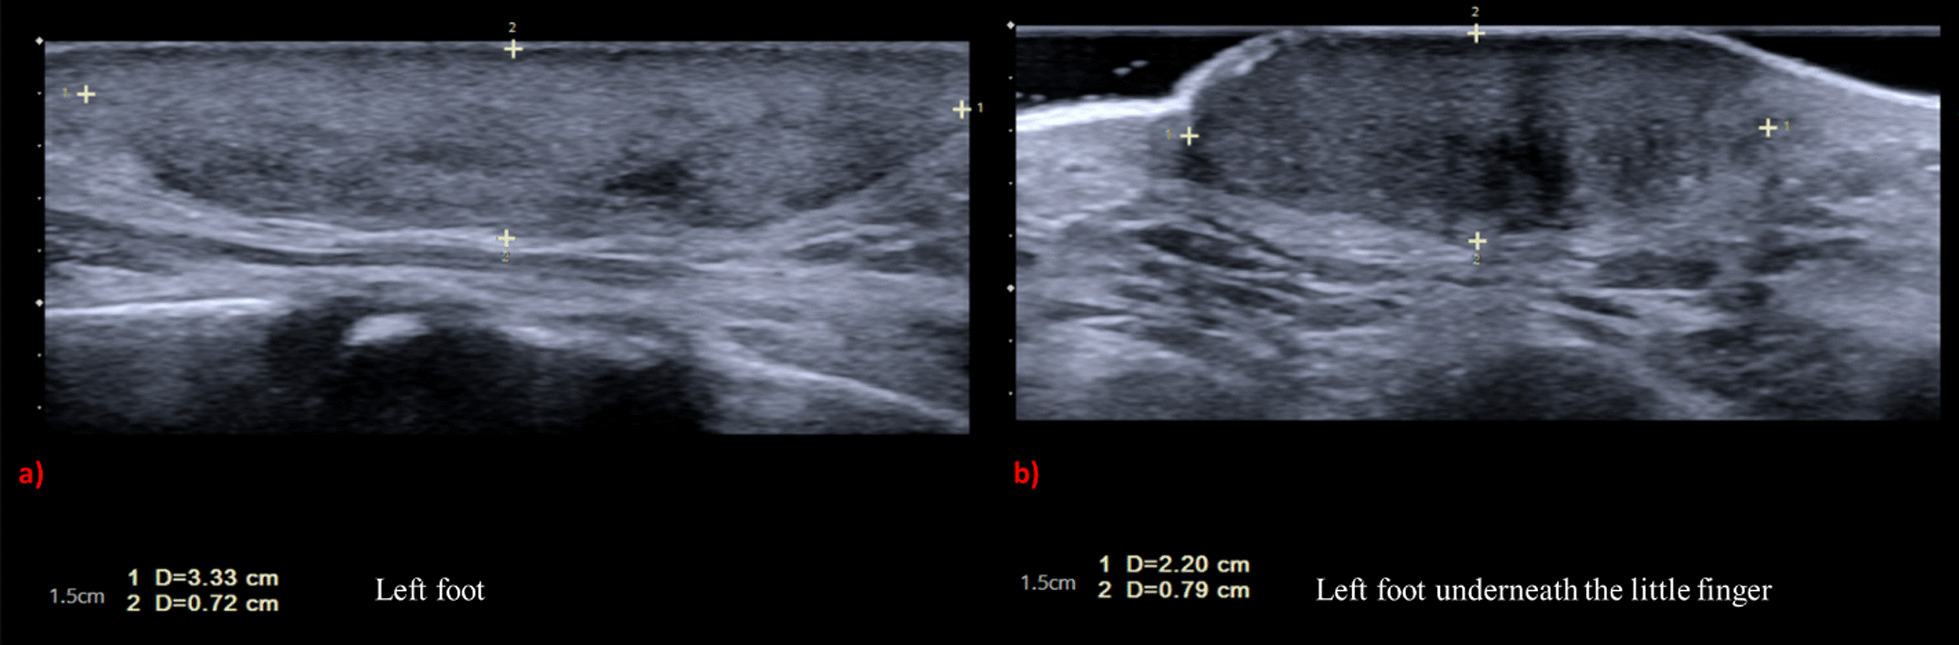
Standard ultrasound scan of the feet showed bilateral multiple fairly well-defined heterogeneously hypoechoic cutaneous/subcutaneous nodules. The largest nodule is seen around the left ankle joint, which measures 3.33 × 0.72 cm (a). The similar smaller lesion seen in the dorsum of the left measures 2.20 × 0.79 cm, with no evidence of extension to deep structures and no internal vascularity or calcifications (b). These nodules are of non-specific sonographic appearance
